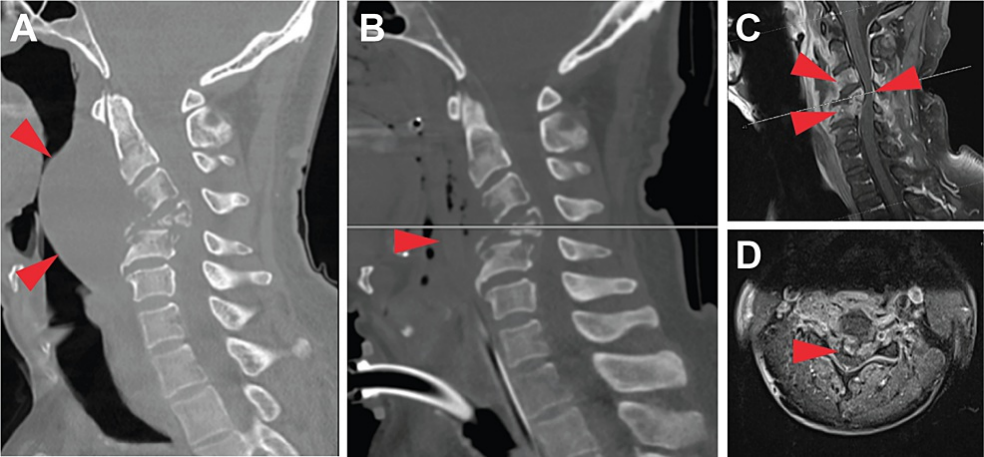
CT and MRI findings during the initial workup and management. (A) Sagittal CT demonstrates sizeable prevertebral collection. Posteriorly, there is cervical spinal canal stenosis due to extensive vertebral body destruction and retropulsion. (B) CT following tracheostomy and pharyngotomy shows a substantial decrease in the size of the prevertebral collection. (C and D) Sagittal and axial post-contrast MRI highlights extensive vertebral body enhancement, bony destruction, cervical spinal stenosis, and spinal cord compression. CT, computed tomography; MRI, magnetic resonance imaging
